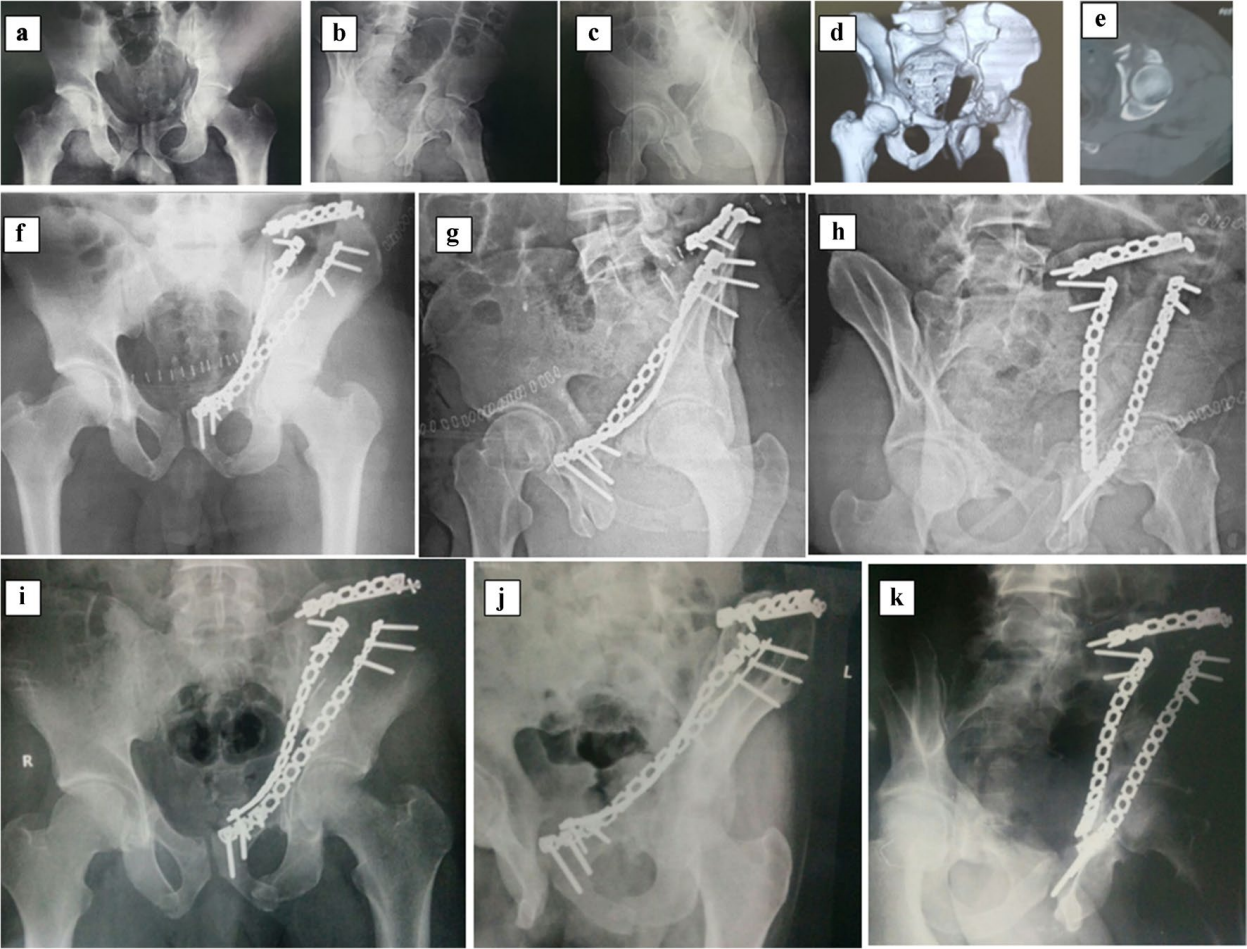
A 36-year-old RTA. a-e preoperative radiographs and computed tomography (CT) scan showing Left Associated Both Column fracture acetabulum. The f–h postoperative radiographs demonstrate the fixation via supra- and infra-pectineal plating and Iliac wing plate, while the i-k radiographs, taken 14 months postoperatively
